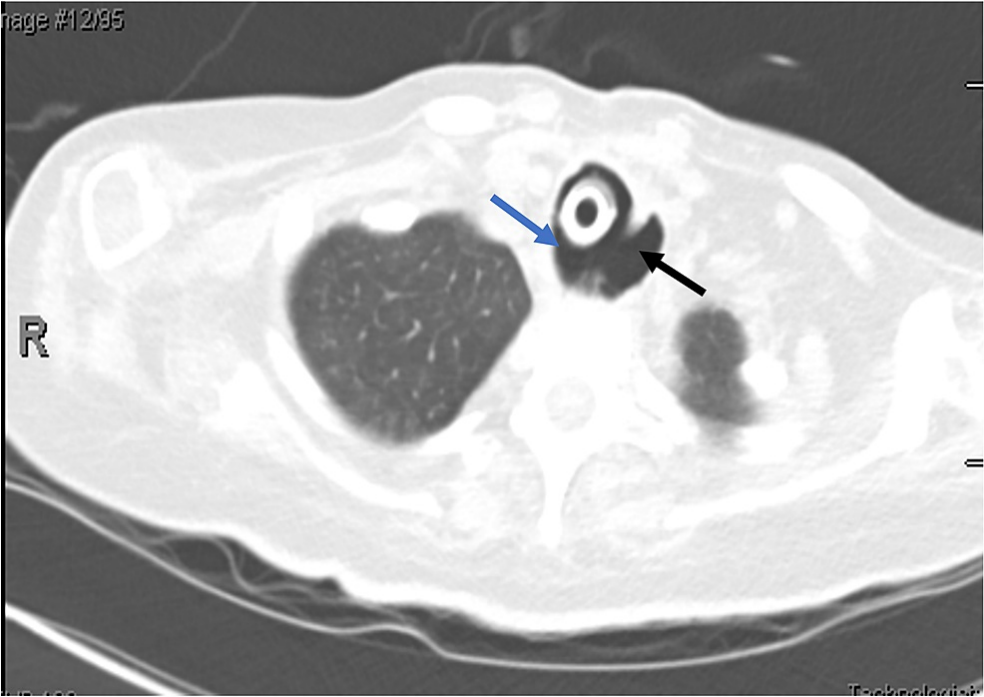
Tracheoesophageal fistula on chest CT. CT scan of the chest reveals tracheoesophageal fistula (blue arrow: trachea, black arrow: esophagus). CT: computed tomography.
